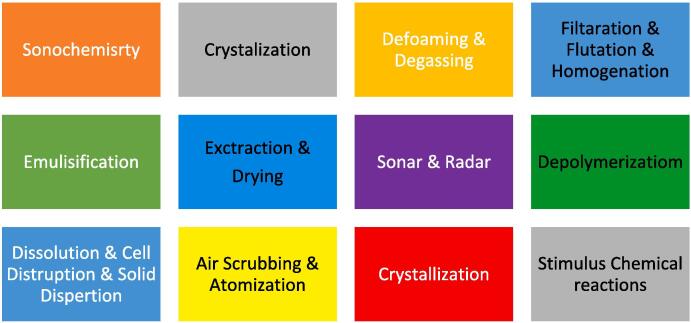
General application of ultrasound.
